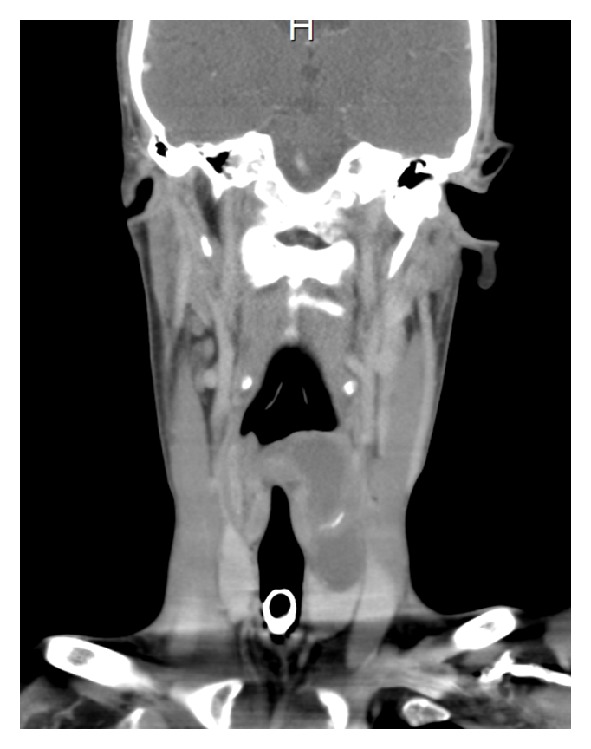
CT scan head and neck, coronal view, well circumscribed mass arising from the left supraglottic larynx extending into the left thyroid lobe.
